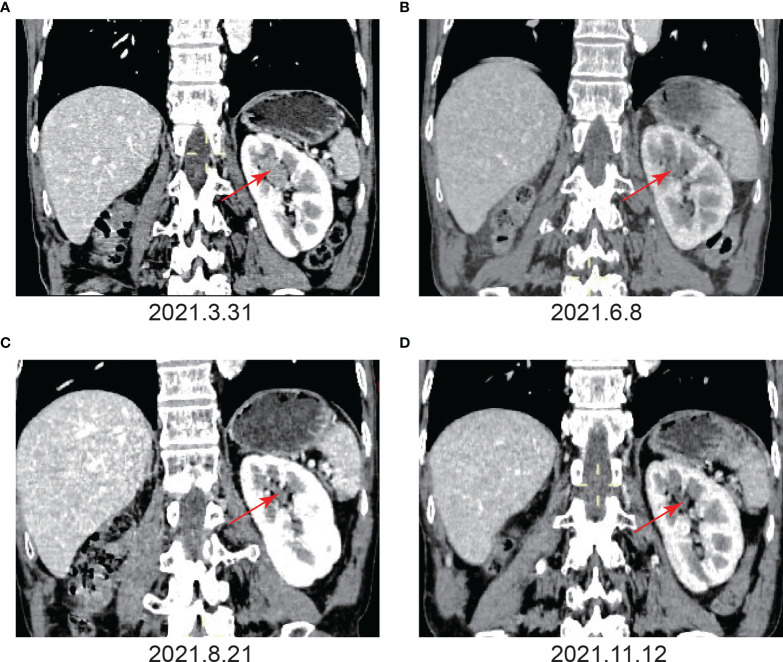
Imaging examination before and after immunotherapy. (A) Before immunotherapy. Enhanced CT showed the absence of the right kidney, a space-occupying lesion in the upper calyx of the left kidney, approximately 27 mm×22 mm×13 mm, uniform density, partly with the renal medulla unclearly, and the enhanced scan showed obvious continuous enhancement. (B) After the fourth immunotherapy. The space-occupying lesion in the upper calyx of the left kidney was significantly smaller than before, and the size was approximately 12 mm×10 mm. (C) After the seventh immunotherapy. The space-occupying lesion in the upper calyx of the left kidney had shrunk to 4 mm×4 mm, and the enhancement degree of the lesion was significantly lower than before. (D) After the ninth immunotherapy. The space-occupying lesion in the upper calyx of the left kidney had shrunk to 3 mm×4 mm.
